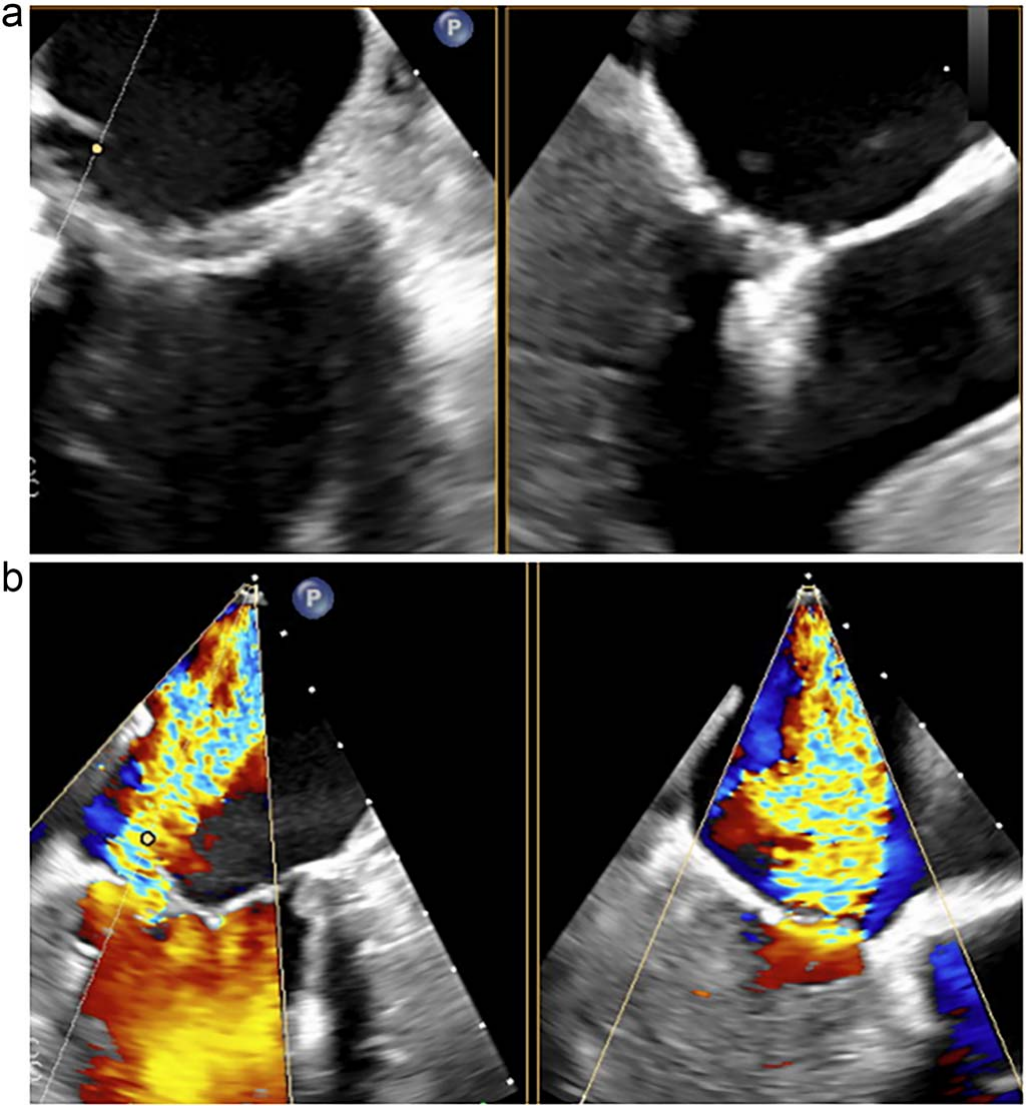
Transesophageal echocardiography following the MitraClip procedure. (a) The posterior mitral leaflet is torn, resulting in a loss of continuity. (b) Mitral regurgitation is observed originating from the site of the tear.
